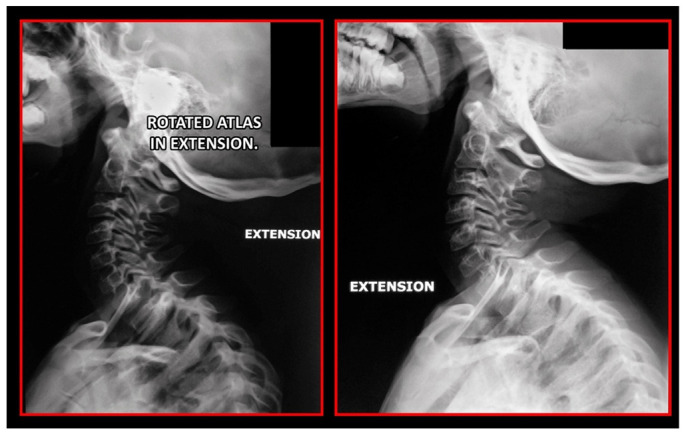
Rotation of the *atlas* on lateral cephalometric radiograph. Left: Pre-treatment. Double ring of the *atlas* showing its rotation. Decreased functional spaces. Right: After orthopedic manual therapy. Only one posterior arch of the *atlas* showing the normalization of *atlas* rotation. Normalized functional spaces.
